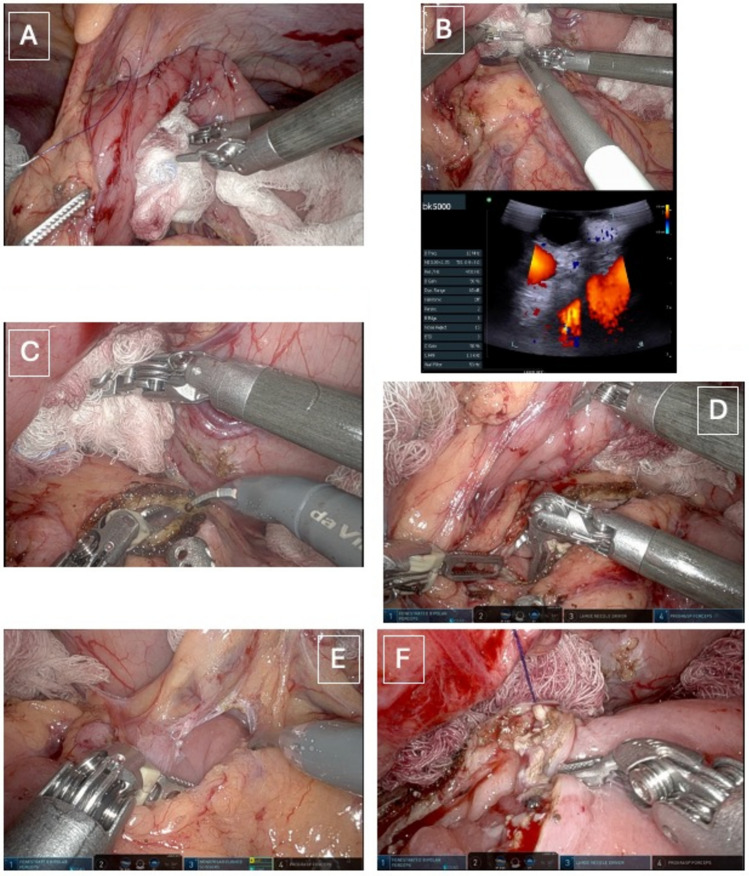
Robotic steps. A stomach is sutured to the anterior abdominal wall for better exposure; B ultrasound to locate the pancreatic duct; C ductotomy using monopolar energy; D exploration of pancreatic duct after ductotomy to remove stones; E opening of transverse mesocolon to create a window for the jejunum to reach the supramesocolic area for the pancreaticojejunal anastomosis; F creation of the pancreaticojejunal anastomosis with VLoc running sutures
